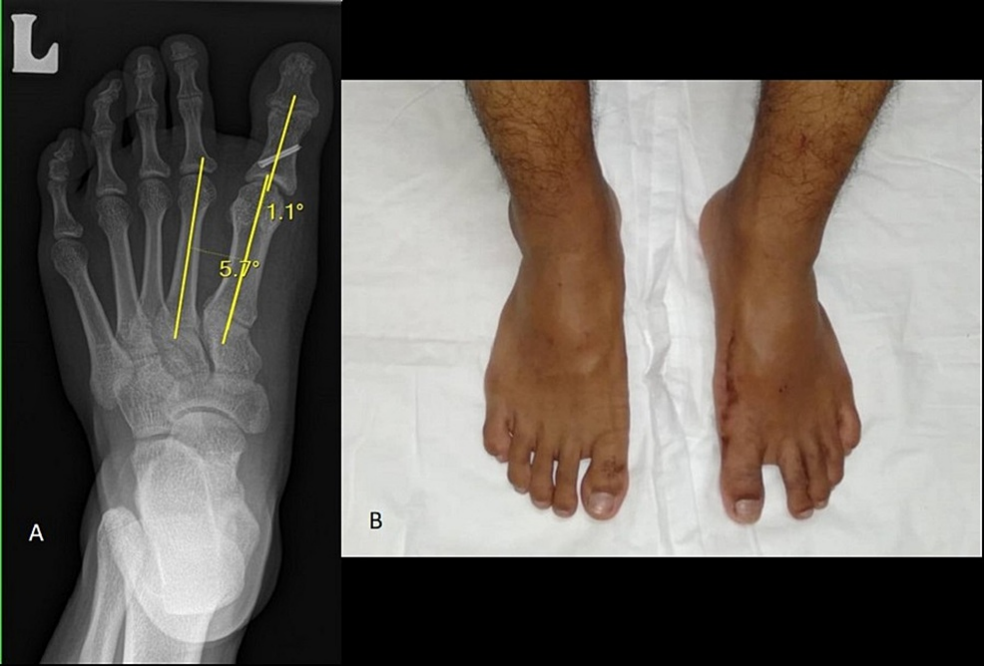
Four-month postoperative X-ray (A) and clinical photo (B) of the left foot showing evidence of union.
